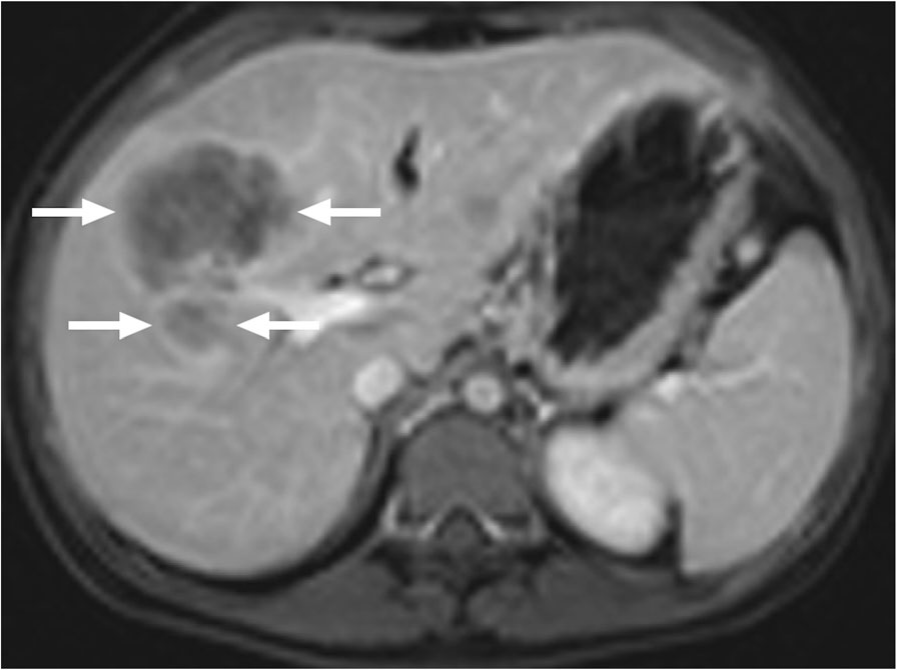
A 16-year-old girl with known surgically treated Wilms tumor undergoing MRI evaluation for newly detected hypoechoic liver mass on US. Axial plane postcontrast T1W MR image demonstrates two peripherally enhancing lesions (arrows) in the right liver lobe. Post percutaneous biopsy showed fascioliasis with no evidence of malignant cells
